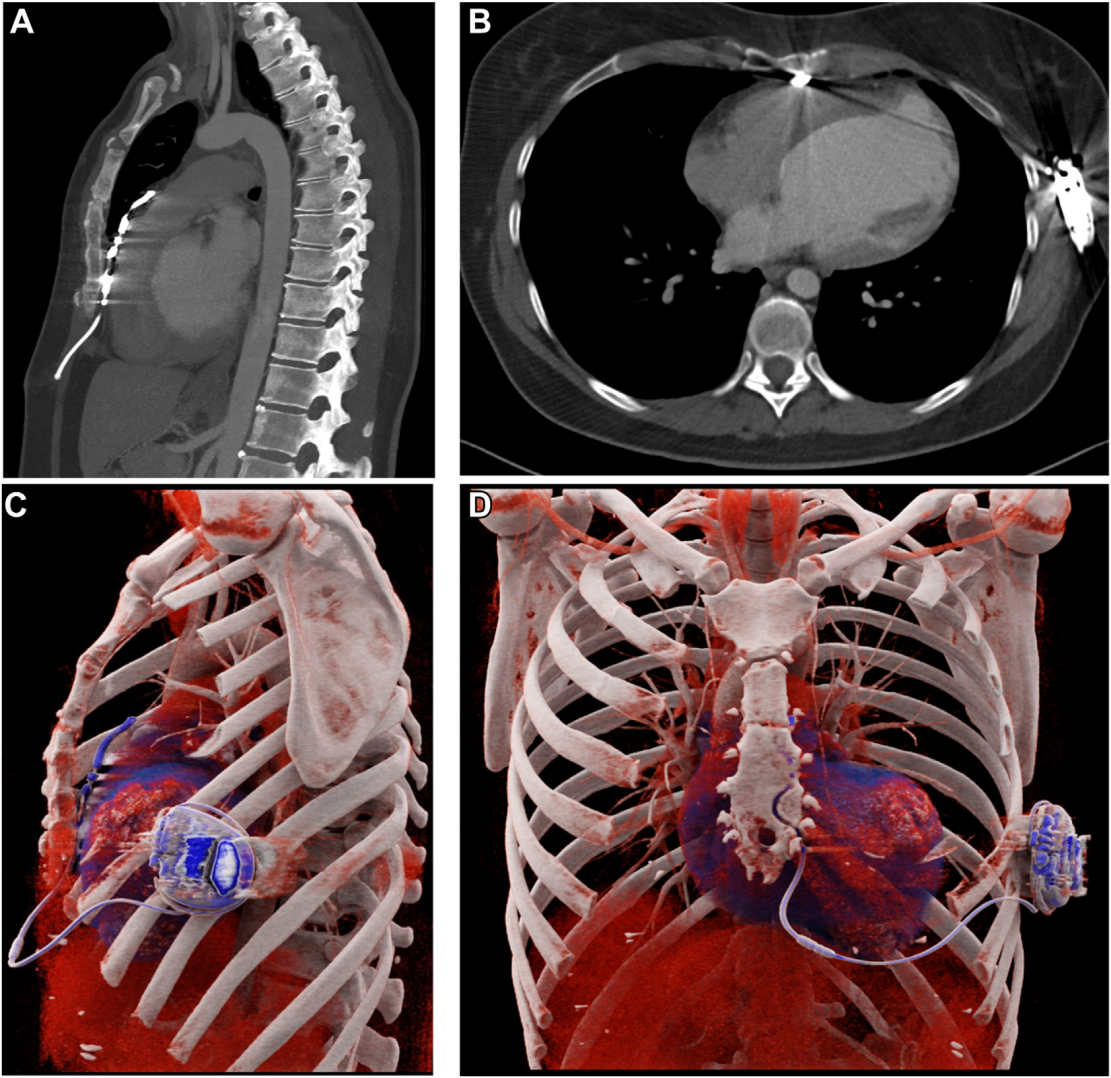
Postoperative computed tomography (CT) of case 1. The CT images (A, B) and 3-dimensional (3D) reconstruction (C, D) show posterior tilting of the extravascular implantable cardioverter-defibrillator lead toward the heart. The ribs were partly removed from the 3D reconstruction to visualize the position of the device. Image B shows the relatively narrow space between the inferior part of sternum and the cardiac structures can be seen. The retrosternal space is larger at the level of middle and superior one-third of the sternum. Images A and B show the streak-like, beam-hardening artifacts induced by the metallic pacing lead.
